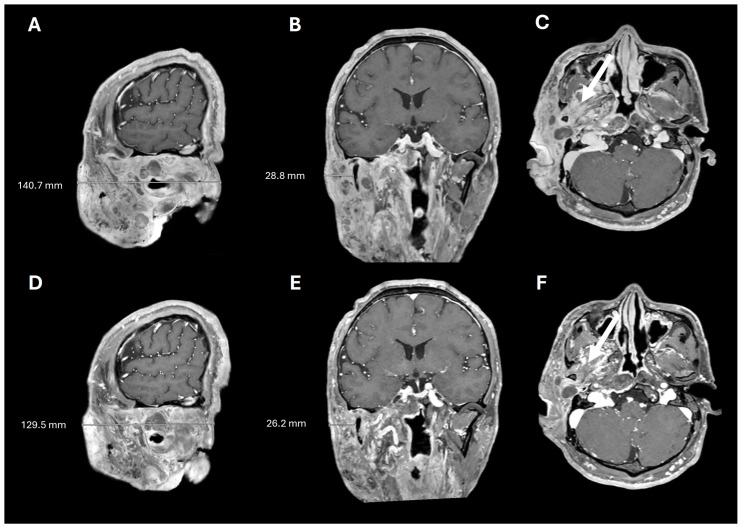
Pre- and post-selumetinib brain MRI. T1+contrast imaging before (A–C) and after (D–F) 7 months of selumetinib, showing decreased tumor burden and decreased tumor infiltration into the masticator space (arrows).
